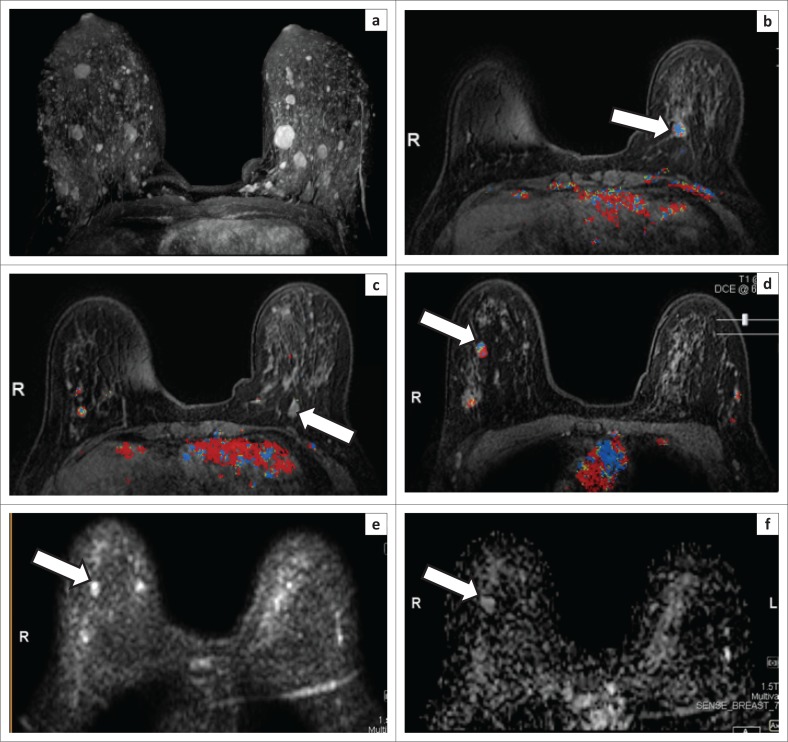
(a) A maximum intensity projection image of the T1-weighted fat saturation post-contrast sequence of a patient with a proven intermediate phyllodes tumour in the right breast demonstrating multiple bilateral breast lesions. (b–d) The dynamic contrast-enhanced images of the same patient demonstrating multiple lesions with varying enhancement patterns. The superimposed colour map on each image reflects the enhancement curves of the pixels. Pixels in red indicate areas with a washout curve, green a plateau curve and blue a persistent enhancement curve. The lesion demonstrated with an arrow in (b) shows a typically persistent enhancement curve. The lesion indicated with an arrow in (c) did not demonstrate any enhancement. The lesion shown with an arrow in (d) had a predominant washout curve and was proven to be a phyllodes tumour. (e) Diffusion-weighted imaging demonstrating restricted diffusion in the phyllodes tumour (arrow). (f) The apparent diffusion coefficient (ADC) map for the phyllodes tumour (arrow) demonstrated a low ADC value of 1.33 mm^2^/s.
